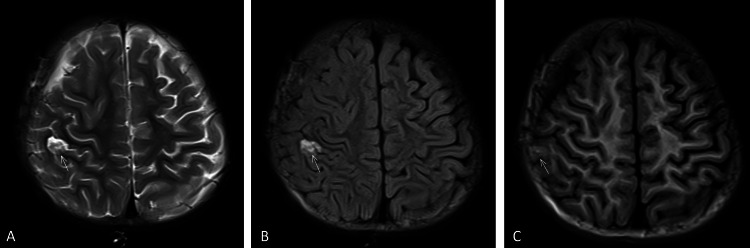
Characterization of the artifact in Case 2 (A) Axial T2 and (B) axial T2FLAIR reveal an oval-shaped uneven hyperintense artifact in the right parietal lobe (arrows). The anterior margin is relatively well-demarcated, while the posterior margin is ill-defined. (C) On Axial T1, a faint, ill-defined patchy hyperintensity (arrow) is observed adjacent to the inner table of the skull, with a lateral displacement compared to its location on T2 and T2FLAIR sequences. FLAIR: Fluid-attenuated inversion recovery.
